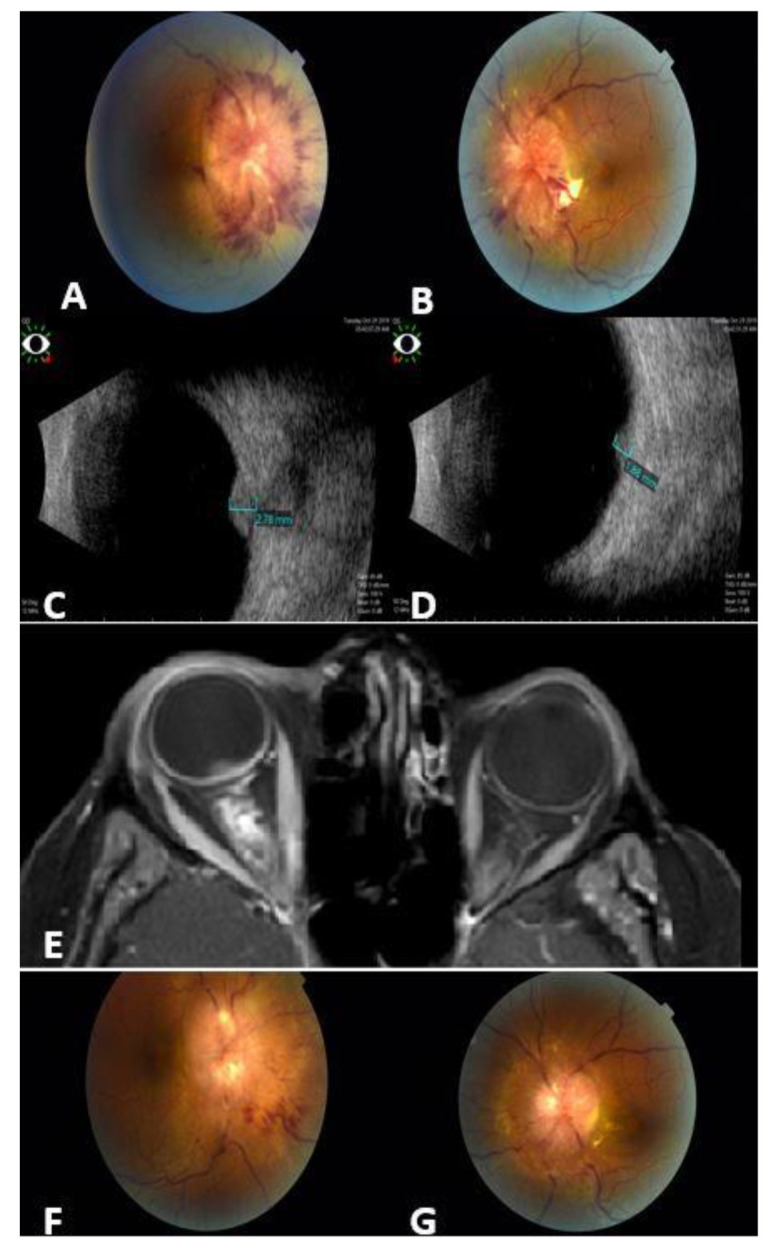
A 43-year-old female with metastatic breast cancer to the optic nerves. Fundus photos for the right (A) and left (B) eyes show bilateral infiltrates around the optic nerves, more prominent in the right eye (A). Ocular B-scan photos show clearly the hyperechoic masses over the right (C) and left (D) optic nerves. Orbit MRI Axial T1-weighted scan with contrast (E) shows thickening of the optic nerve sheath that extends to the optic nerve head (more prominent on the right side), irregular outline, and stranding of surrounding fat planes. Fundus photos for the same patient 3 months post orbit EBRT: major regression in optic nerve infiltrates in both eyes: right (F); left (G).
